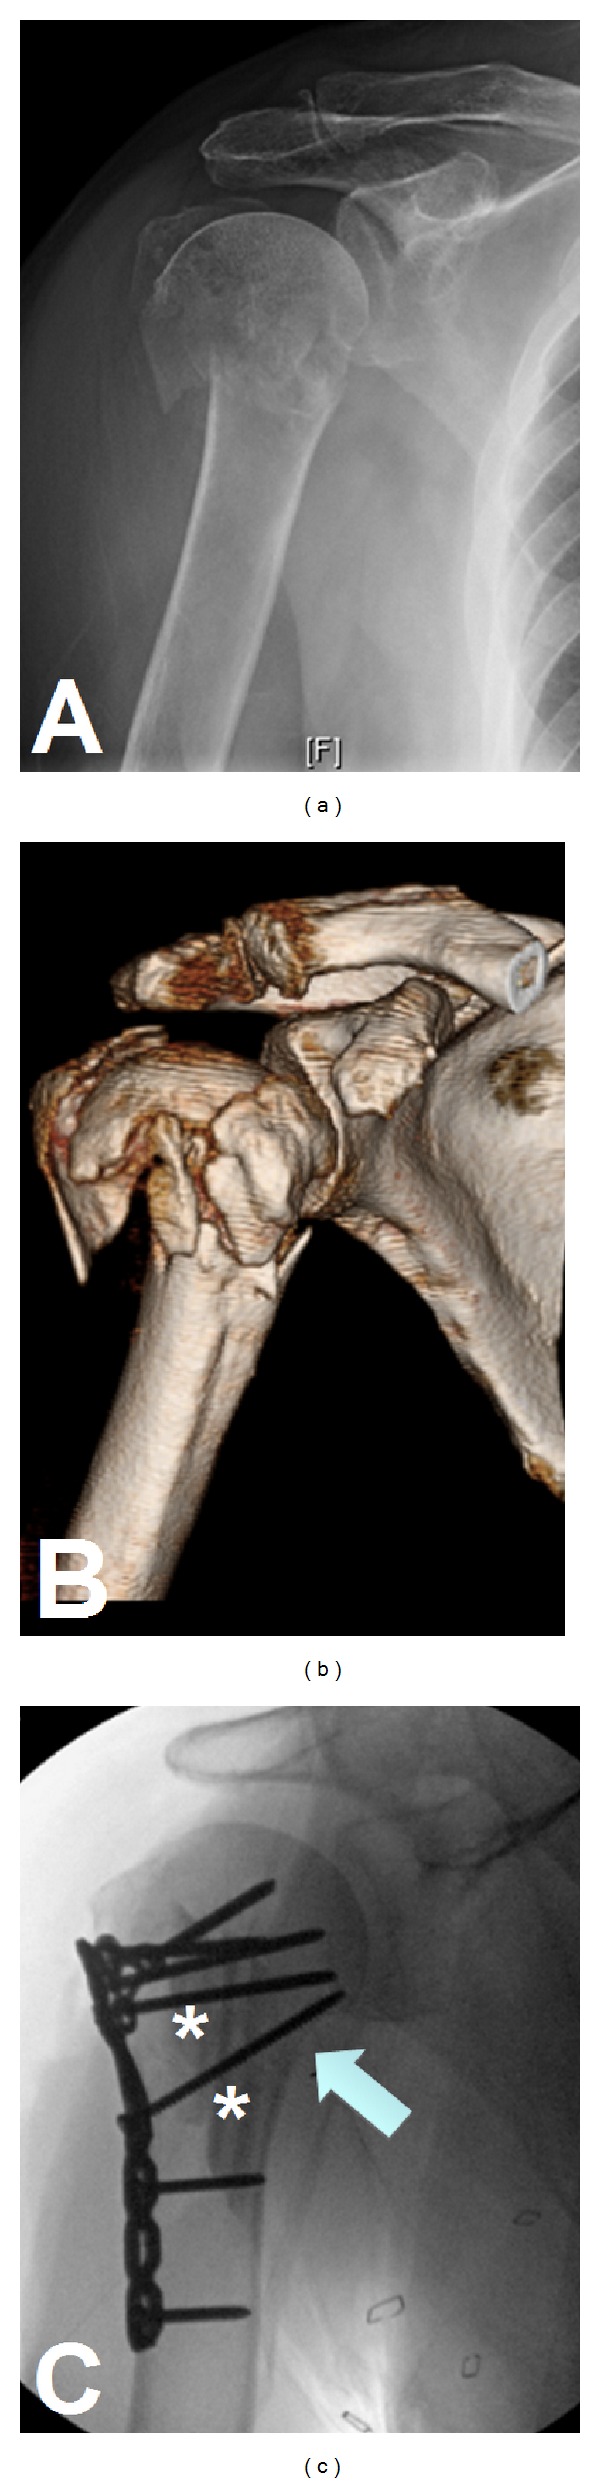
Radiograph (a) of a proximal humerus fracture. The CT scan with 3D reconstruction (b) adds significant detail and aids in preoperative planning. Osteosynthesis was carried out (c) with the use of intramedullary fibulas (asterisks) and particular attention paid to restoration of the medial calcar (arrow).
